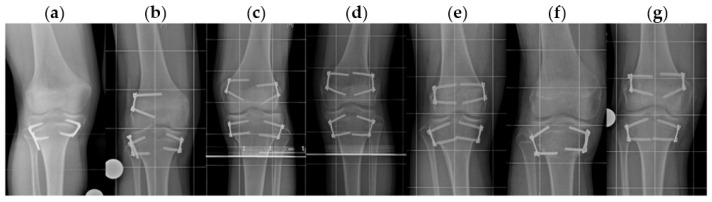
Anteroposterior radiographs of the 7 patients in whom newly occurred proximal fibular overgrowth was observed at the end of treatment (a,b) with (*n* = 2) and (c–g) without temporary proximal fibular epiphysiodesis (*n* = 5).
